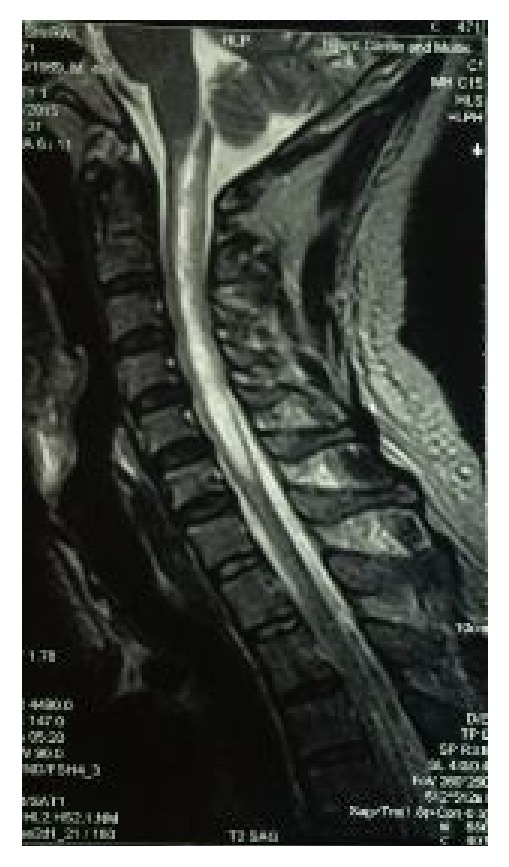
Magnetic resonance imaging of cervical spine; saggittal view of cervical segment of spinal cord is shown. The image shows T2 hyperintense signal in central cervical spinal cord from first to sixth cervical level, showing subtle enhancement and causing expansion of involved cord.
